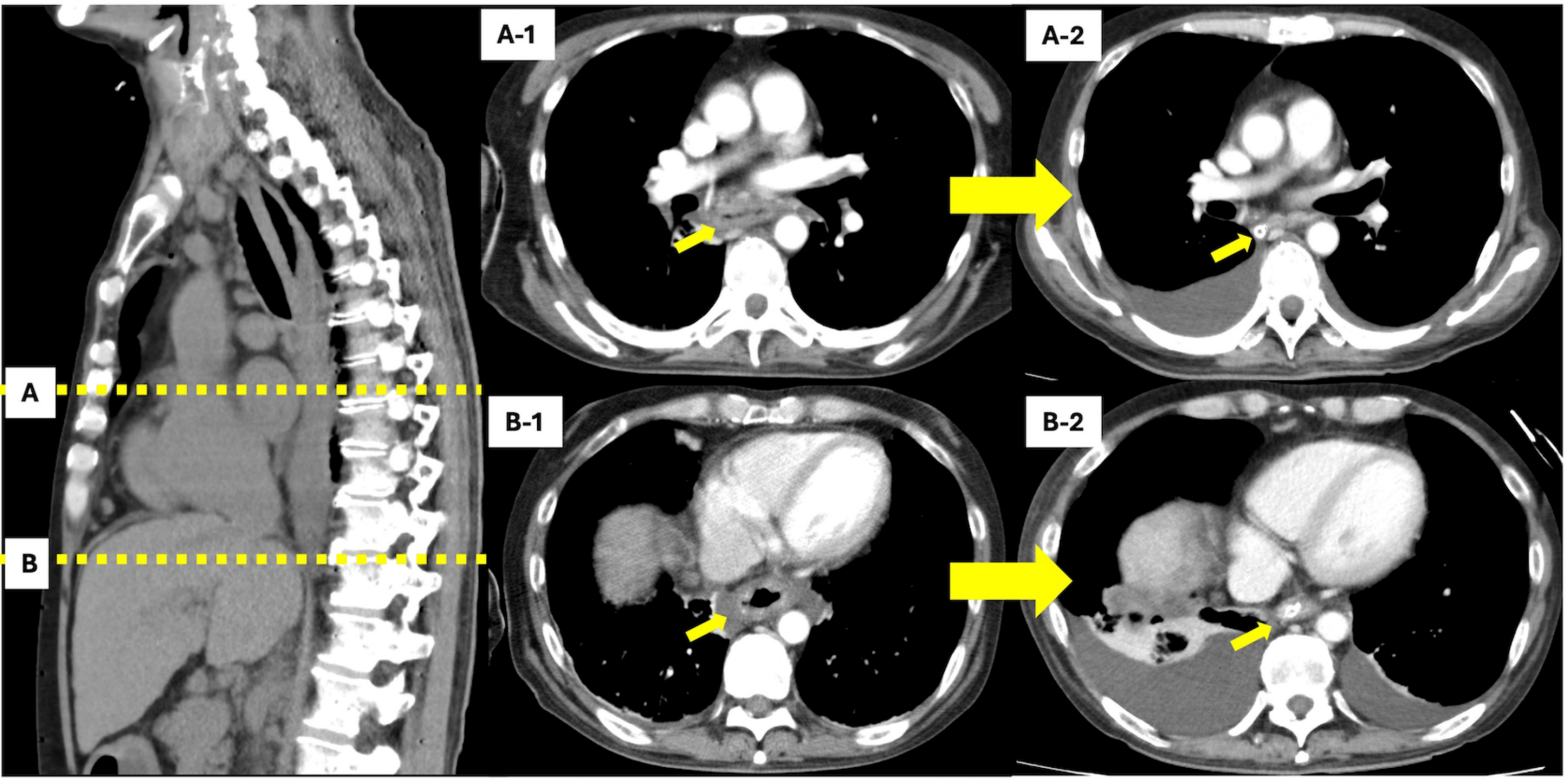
Temporal changes in computed tomography (CT) findings of acute phlegmonous esophagitis A-1 and B-1 show CT images obtained on day 1, revealing esophageal wall thickening and periesophageal inflammation.
